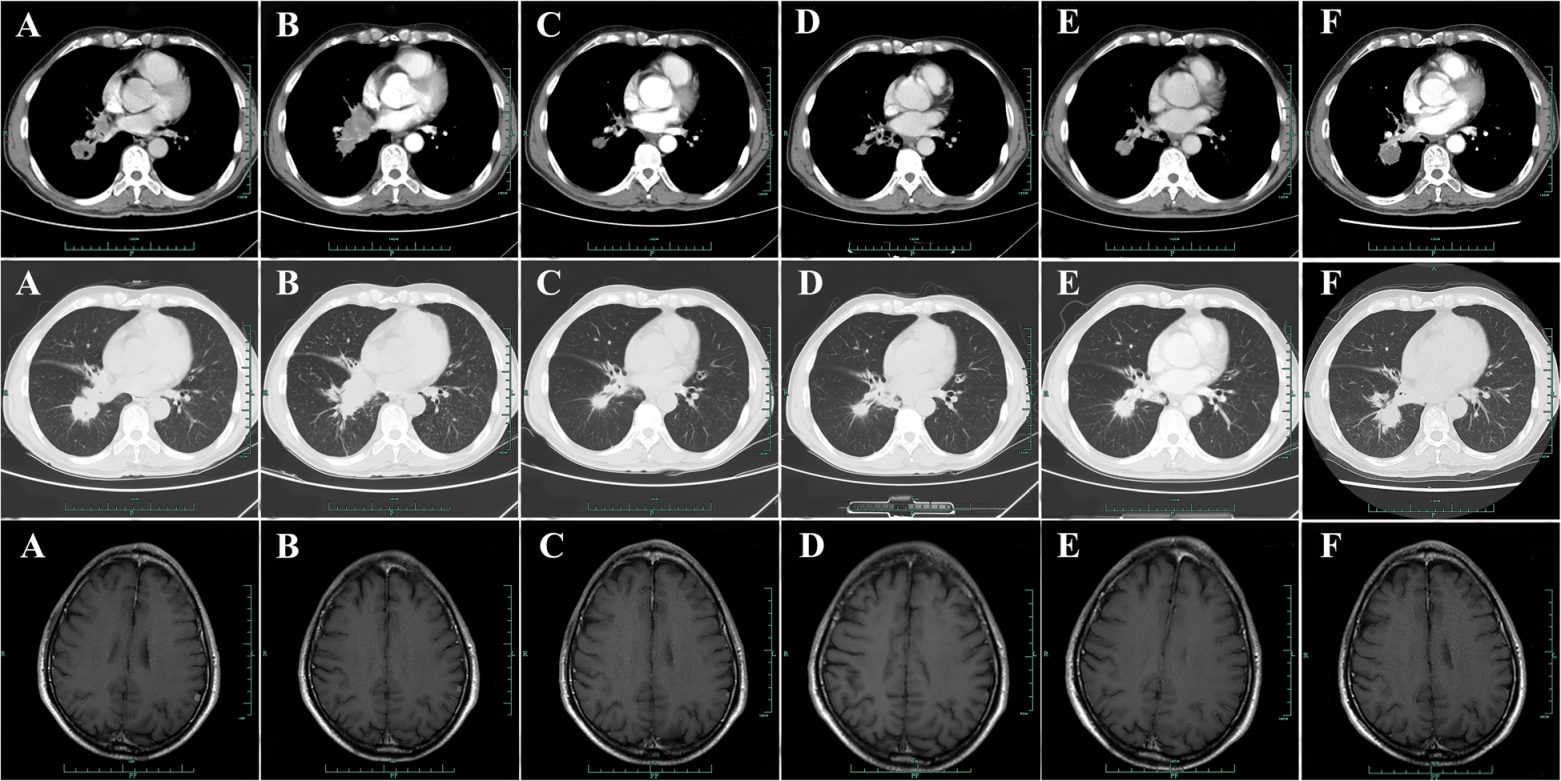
Chest CT scan and brain MRI from April 2015 to February 2016. a Chest CT scan and brain MRI at the time of diagnosis in April 2015. b Chest CT scan and brain MRI after 2 cycles of pemetrexed plus cisplatin treatment in June 2015. c Chest CT scan and brain MRI after 1 month treatment of icotinib in July 2015. d Chest CT scan and brain MRI after 3 months treatment of icotinib in September 2015. e Chest CT scan and brain MRI after 6 months treatment of icotinib in December 2015. f Chest CT scan and brain MRI after 8 months treatment of icotinib in February 2016
